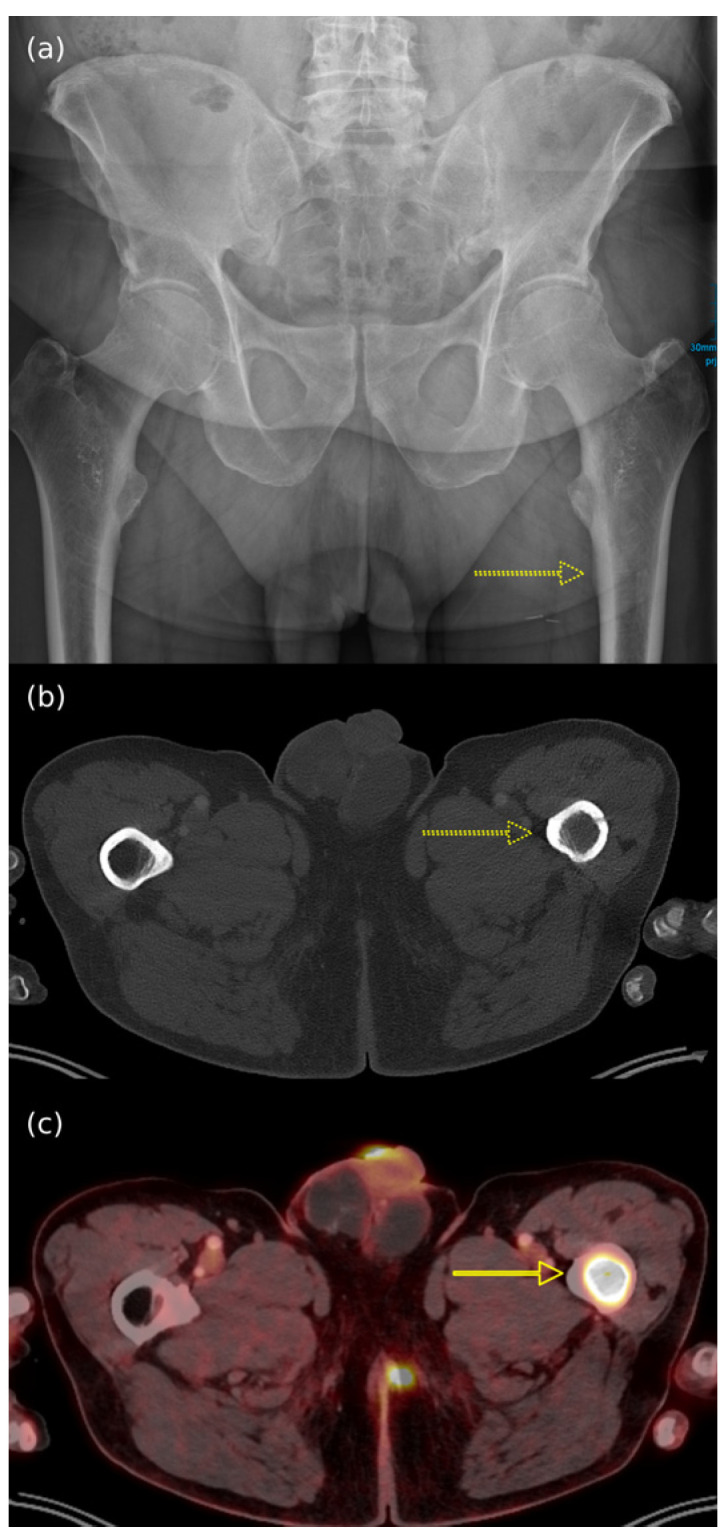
Multimodal imaging of MCC bone metastasis. (a,b) X-ray and CT images of the left femur showing no structural abnormalities (yellow arrows). (c) PET/CT reveals increased metabolic activity in the same region, consistent with bone marrow involvement (yellow arrow).
